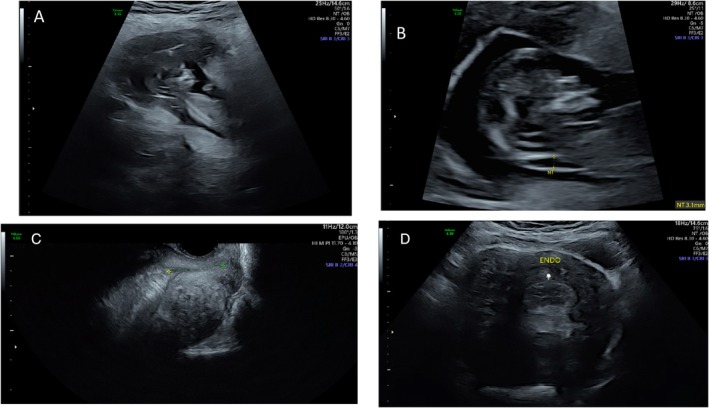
Ultrasound images. (A, B) Initial departmental ultrasound images demonstrating a fetus with a crown‐rump length (CRL) of 69 mm and nuchal translucency of 3.1 mm. The fetal anatomy and amniotic fluid volume was described as normal. The placenta was described as posterior. (C, D) Second ultrasound within early pregnancy unit scan demonstrating empty uterine cavity both transvaginally and transabdominally.
